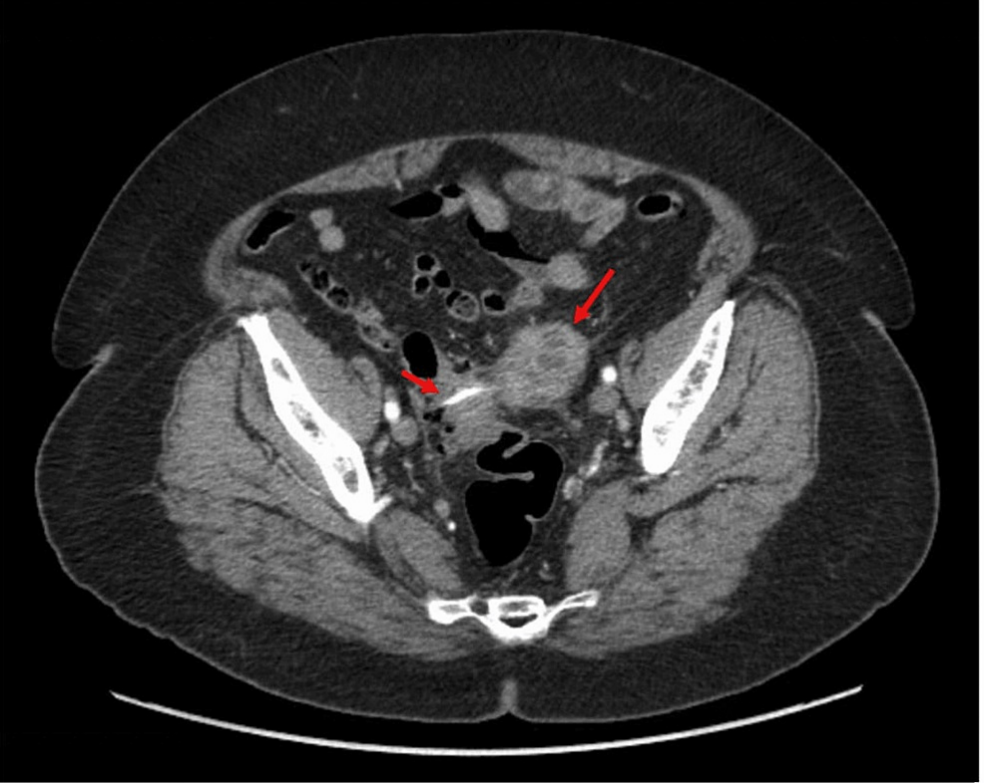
Initial CT abdomen pelvis with contrast image showing a needle-like foreign body with adjacent abdominal abscess measuring 5.0 cm × 4.3 cm × 4.4 cm, as indicated by the arrows. CT, computed tomography
